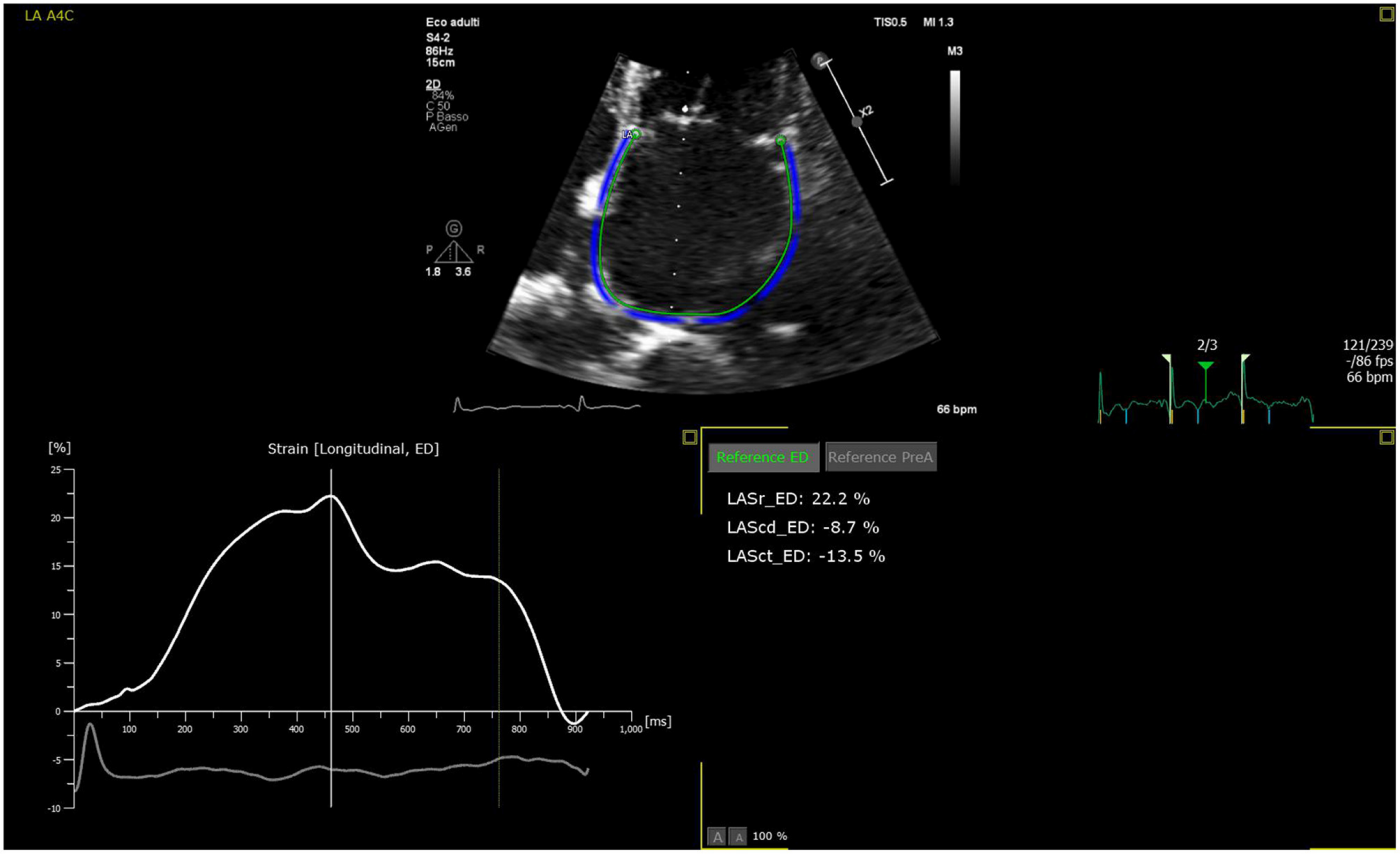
LA strain curve with R-R gating method in apical four-chamber view with zoom-in focus. The patient is an 83-year-old female with chronic heart failure. The patient is in sinus rhythm with reservoir function reduced. Zoomed image is used to increase the frame rate to enhance strain analysis accuracy.
